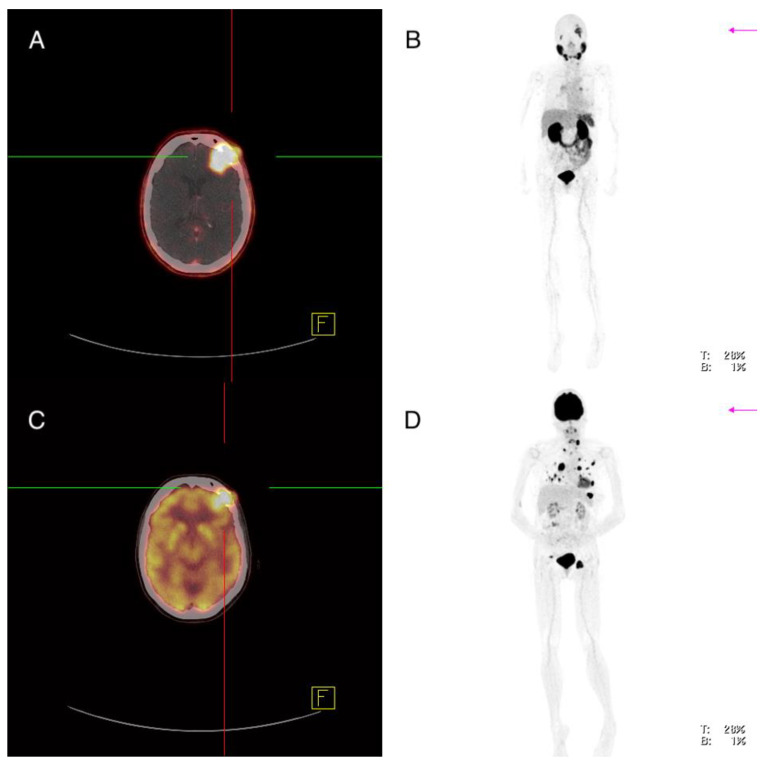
Patient 7: ^68^Ga-PSMA-PET/CT (A,B), ^18^FDG-PET/CT (C,D). A cerebral metastasis left frontal shows increased PSMA expression in concordance to an increased FDG uptake.
